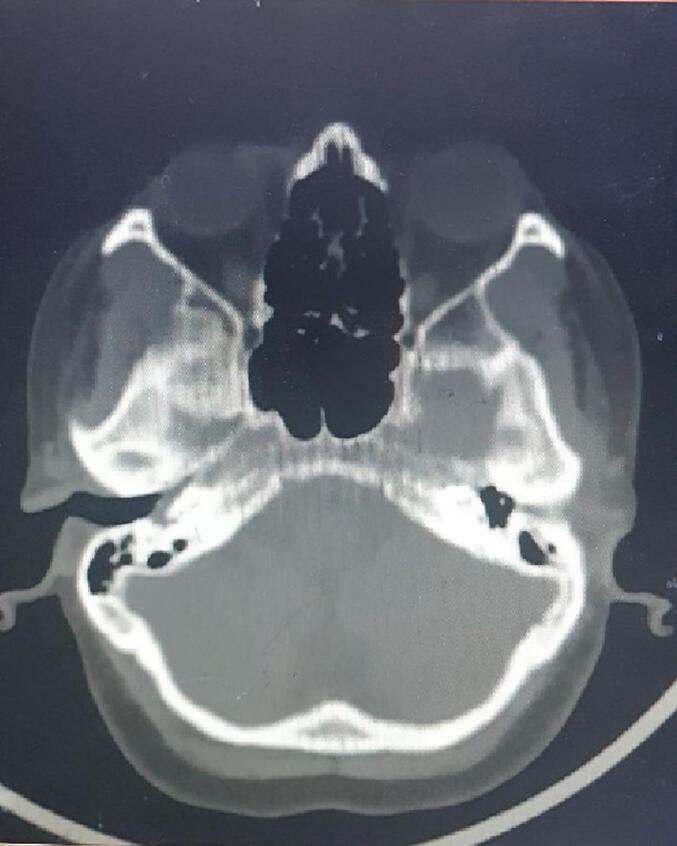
High-resolution computed tomography scan showing a mass on the promontory and occupying the right middle ear.
